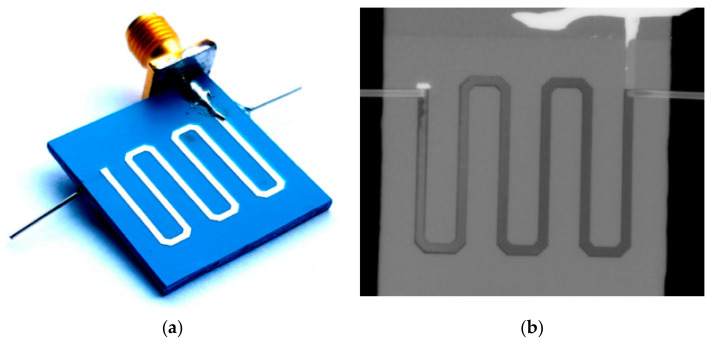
The photo of the Low-Temperature Cofired Ceramic (LTCC) device of heating (a) and the X-ray image with the microchannel placed below the microstrip line (b).
